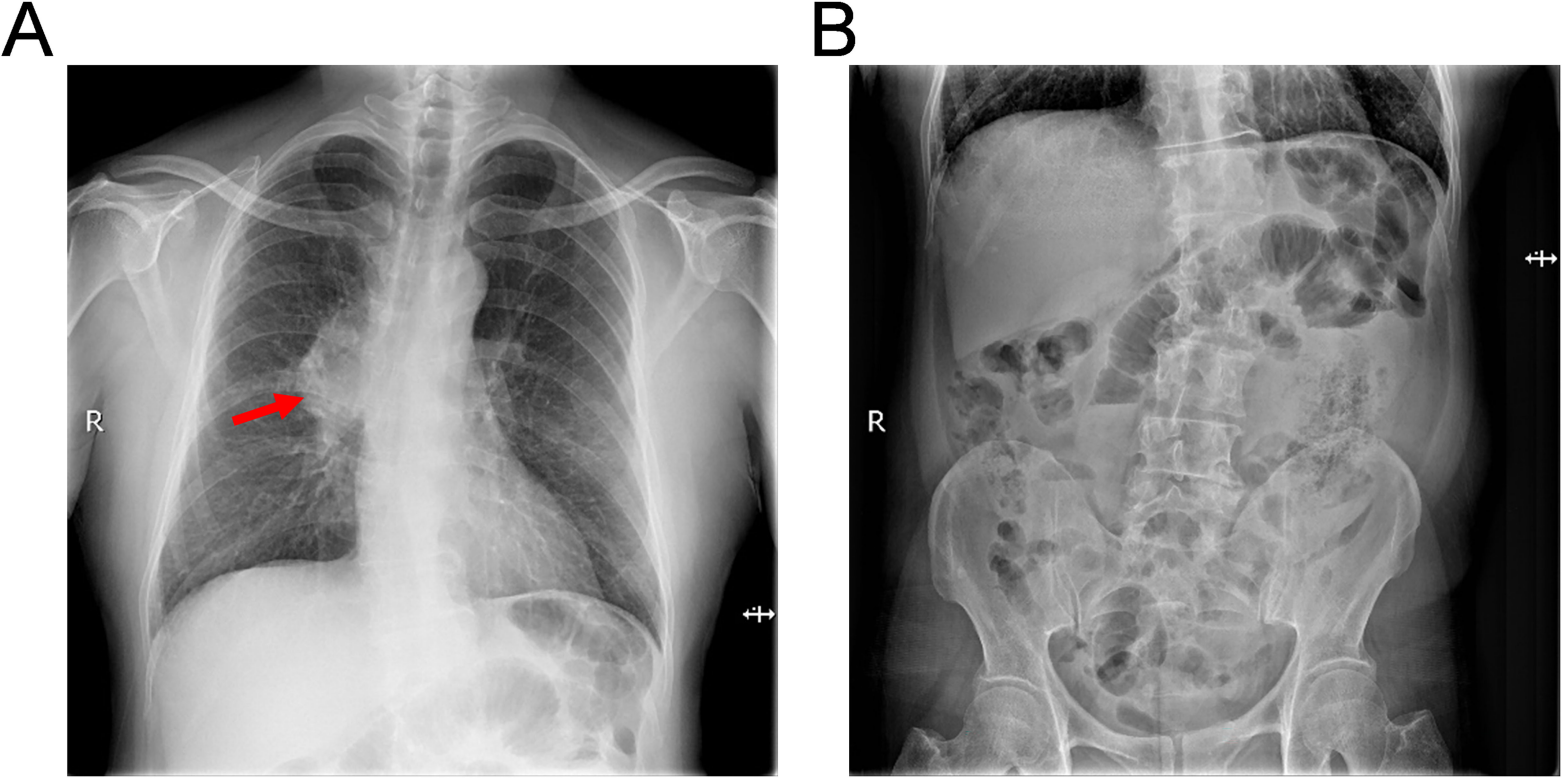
Radiographic assessment of thoracic and abdominal anatomy upon hospital presentation. (A) Thoracic radiography demonstrates bilateral pulmonary patchy opacities, indicative of infiltrative disease, along with pronounced broncho-vascular markings and a dense mass within the right pulmonary hilum (as shown by red arrows), suggesting a bronchial lung cancer or inflammatory process. (B) Abdominal radiography exhibits dilatation of intestine with the presence of air-fluid levels, consistent with the clinical suspicion of an incomplete intestinal obstruction.
